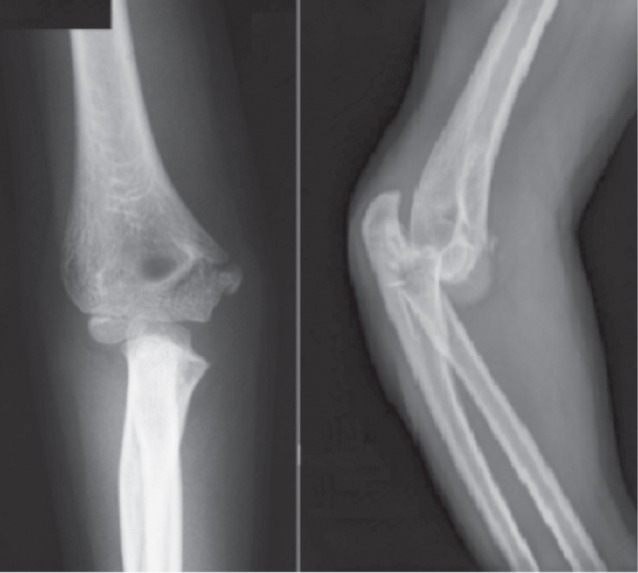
Anteroposterior and lateral radiographs views of the elbow showing a posterior dislocation of the elbow associated to fracture of the medial condyle.
